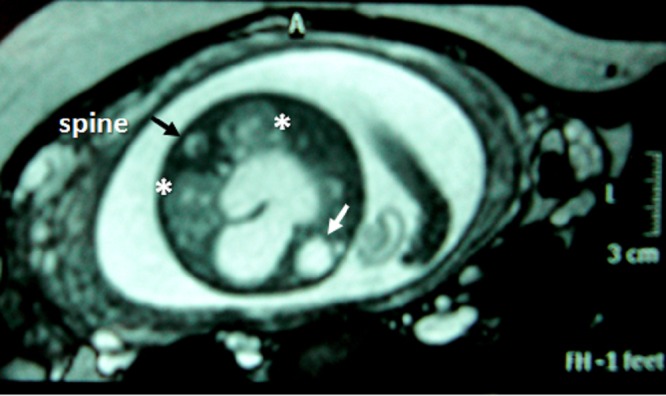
Figure 4: Fetal MRI axial plane.
